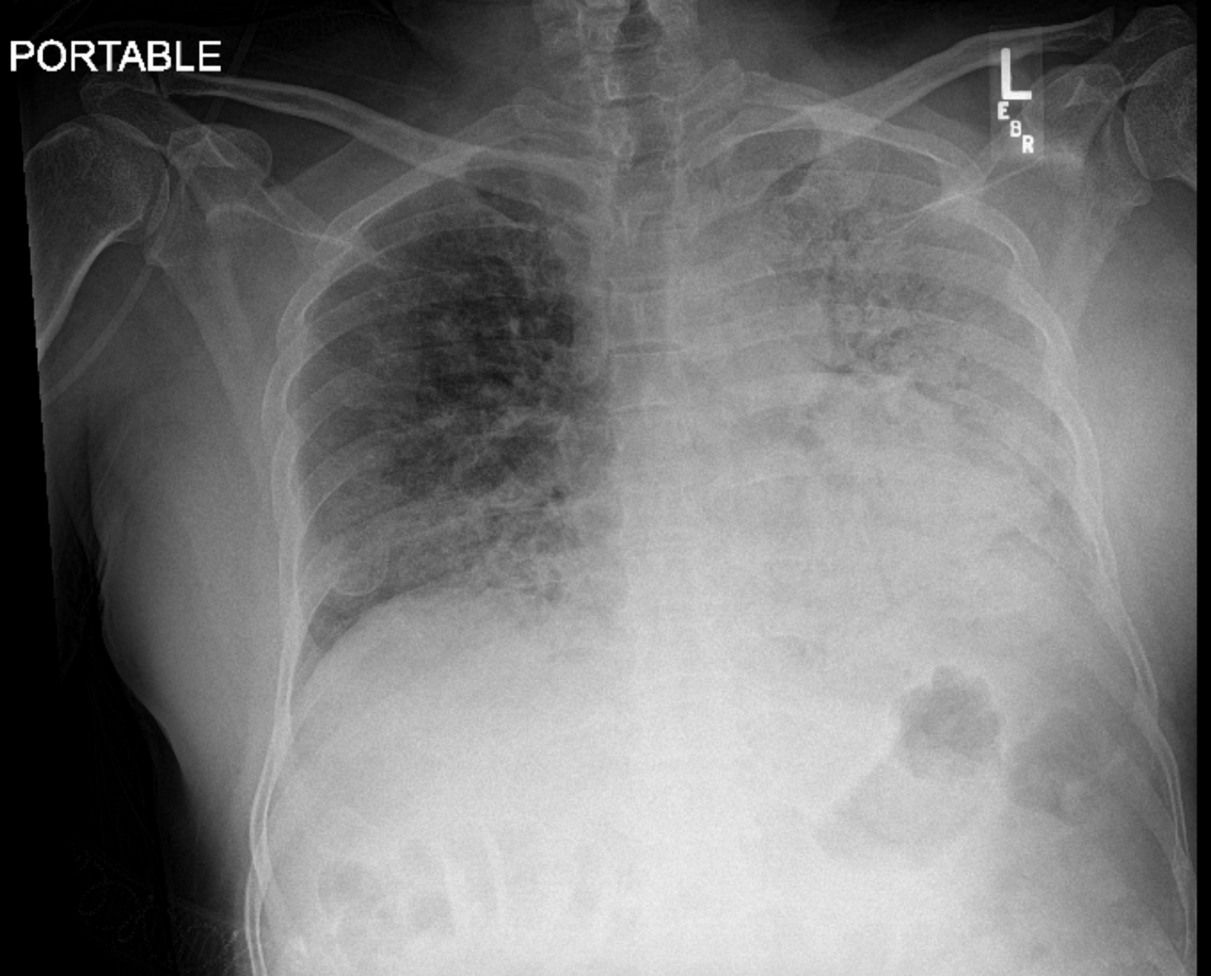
Portable AP chest X-ray demonstrating pan lobar infiltrates with more confluent opacity in the lower lungs, worse on the left than the right. AP, anteroposterior
